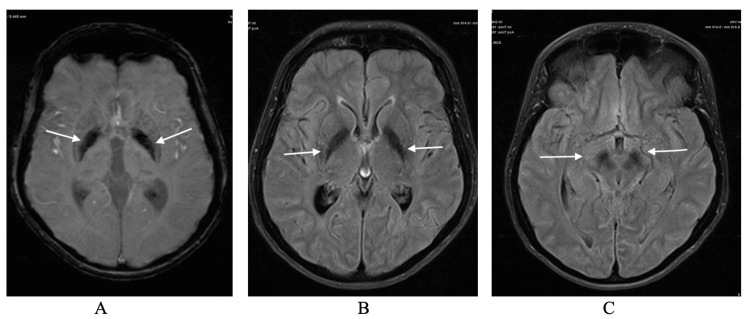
Image A is showing blooming artifact on Susceptibility Weighted Images (SWI) representing iron depositions involving the globus pallidus bilaterally. Images B and C are demonstrating low signal intensity on T2-weighted images involving the globus pallidus bilaterally
